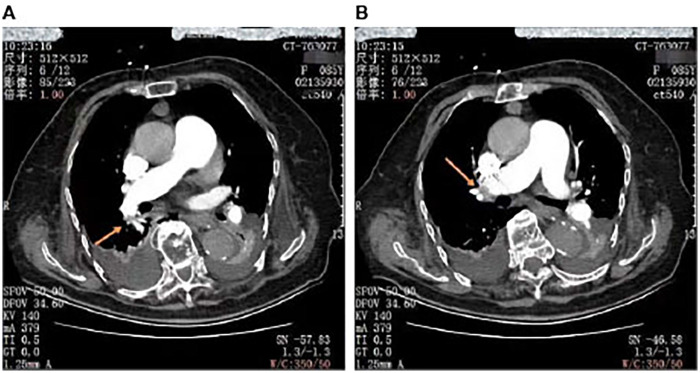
Computed tomography pulmonary angiogram (CTPA) revealing pulmonary embolism in the right upper and lower lobes, associated with bilateral pleural effusion and dependent atelectasis (August 28, 2024). A. Arrow indicates: Axial view of pulmonary embolism in the right lower lobe pulmonary artery. B. Arrow indicates: Axial view of pulmonary embolism in the right main pulmonary artery.
